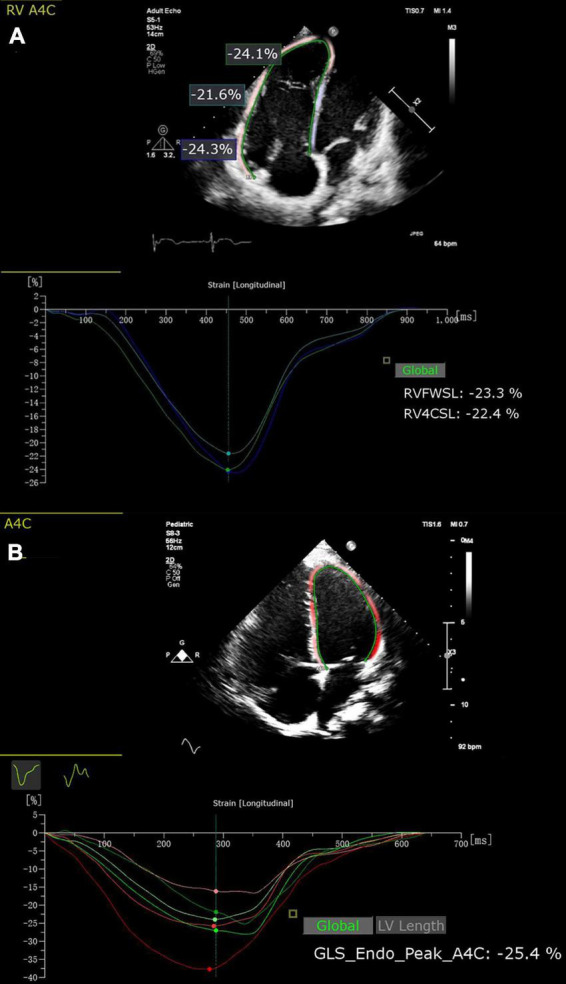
Two-dimensional speckle tracking echocardiography (2D-STE) offline analysis for RVFWLS (A), RVGLS (A) and LVGLS (B). RVFWSL, right ventricular free wall longitudinal strain; RV4CSL, right ventricular global longitudinal strain.
